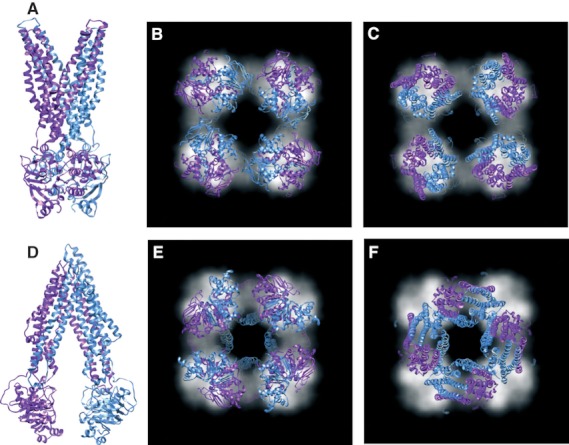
Fits of ABC X-ray structures into the EM maps. (A) Structure of the Sav1866 homodimer (one monomer is coloured blue and the other purple). (B, C) X-ray structure of Sav1866 homodimer viewed (B) from the intracellular side (hence NBDs are to the front) and (C) from the extracellular side (i.e. TMDs face the front). The 2D EM projection map of SUR2B was positioned at the NBD–TMD interface, in the fitted *x*, *y* orientation, in order to visualize the overlay with the NBDs (B) or the TMDs (C) of the 3D Sav1866 structure (see Fig. 4). The arrow indicates the difference in viewpoint between (B) and (C, D) Structure of P-glycoprotein (TMD1–NBD1 is coloured blue, TMD2–NBD2 purple). (E, F) X-ray structure of P-glycoprotein viewed (E) from the intracellular side (hence NBDs are to the front) and (F) from the extracellular side (i.e. TMDs face the front). The 2D EM projection map of SUR2B was positioned at the NBD–TMD interface, in the fitted *x*, *y* orientation, in order to visualize the overlay with the NBDs (E) or the TMDs (F) of the 3D P-glycoprotein structure (see Fig. 4). The arrow indicates the difference in viewpoint between (E) and (F).
